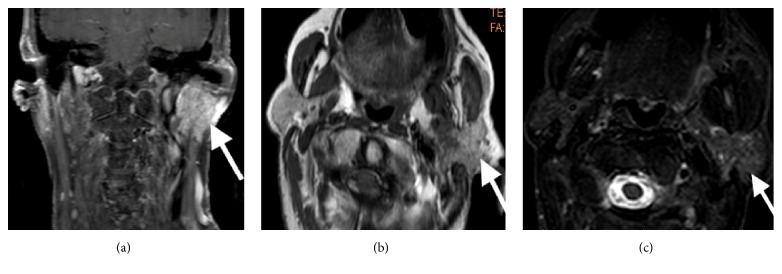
MRI revealing a mass in the deep lobe of the parotid gland abutting the posterior aspect of the lateral pterygoid muscle, likely extending towards the stylomastoid foramen exhibiting low T1 signal intensity in (a) coronal and (b) axial planes as well as low STIR signal intensity in (c) axial plane.
